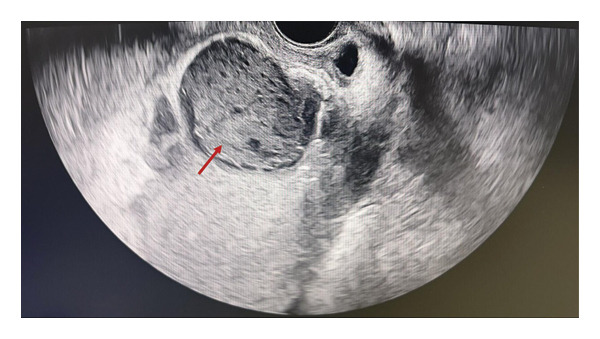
Transvaginal ultrasonography during her presentation. Pelvic fluid collection measuring 55 × 35 mm (red arrow) was found between the uterus and the urinary bladder.
